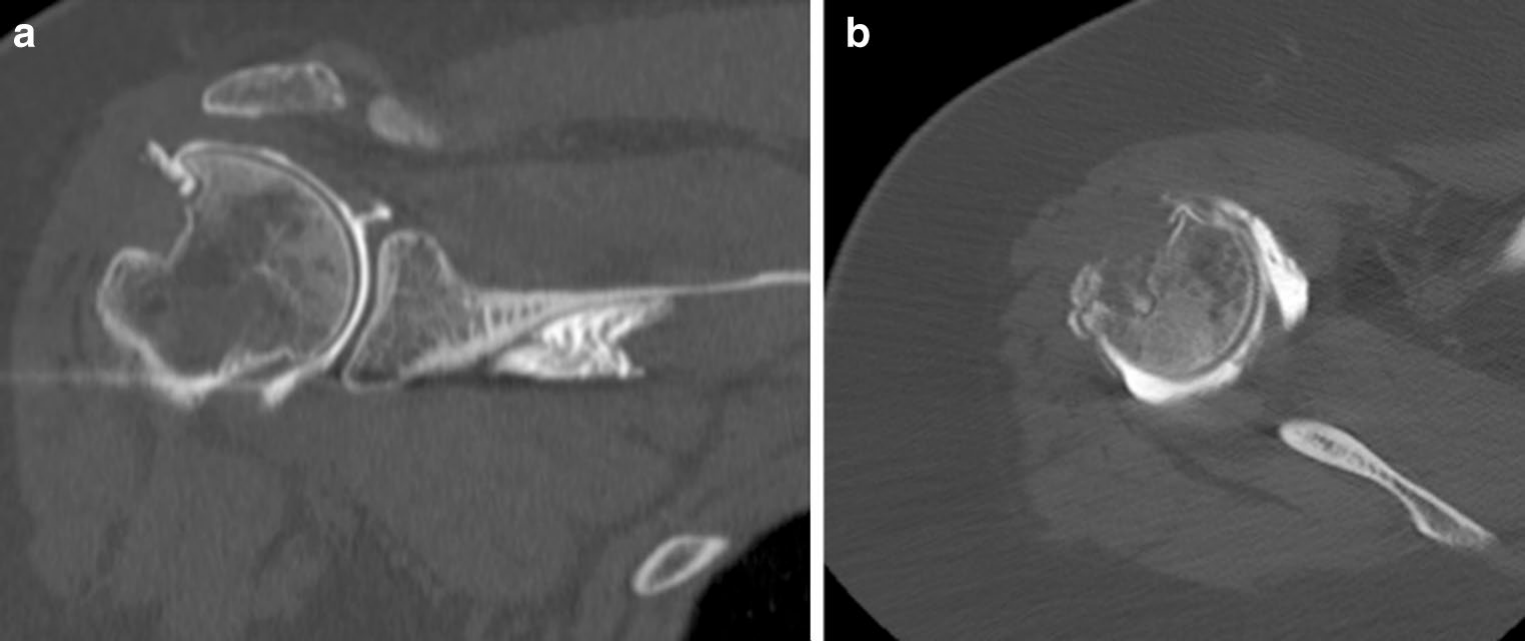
Preoperative coronal (a) and axial (b) CT scan demonstrating massive greater tuberosity bone loss
